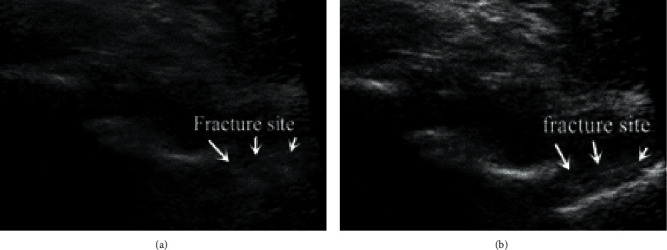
Comparison of scapula fracture before and after ultrasound artificial intelligence enhancement. (a) Unenhanced ultrasound image of the scapula. (b) Enhanced ultrasound image of the scapula.
